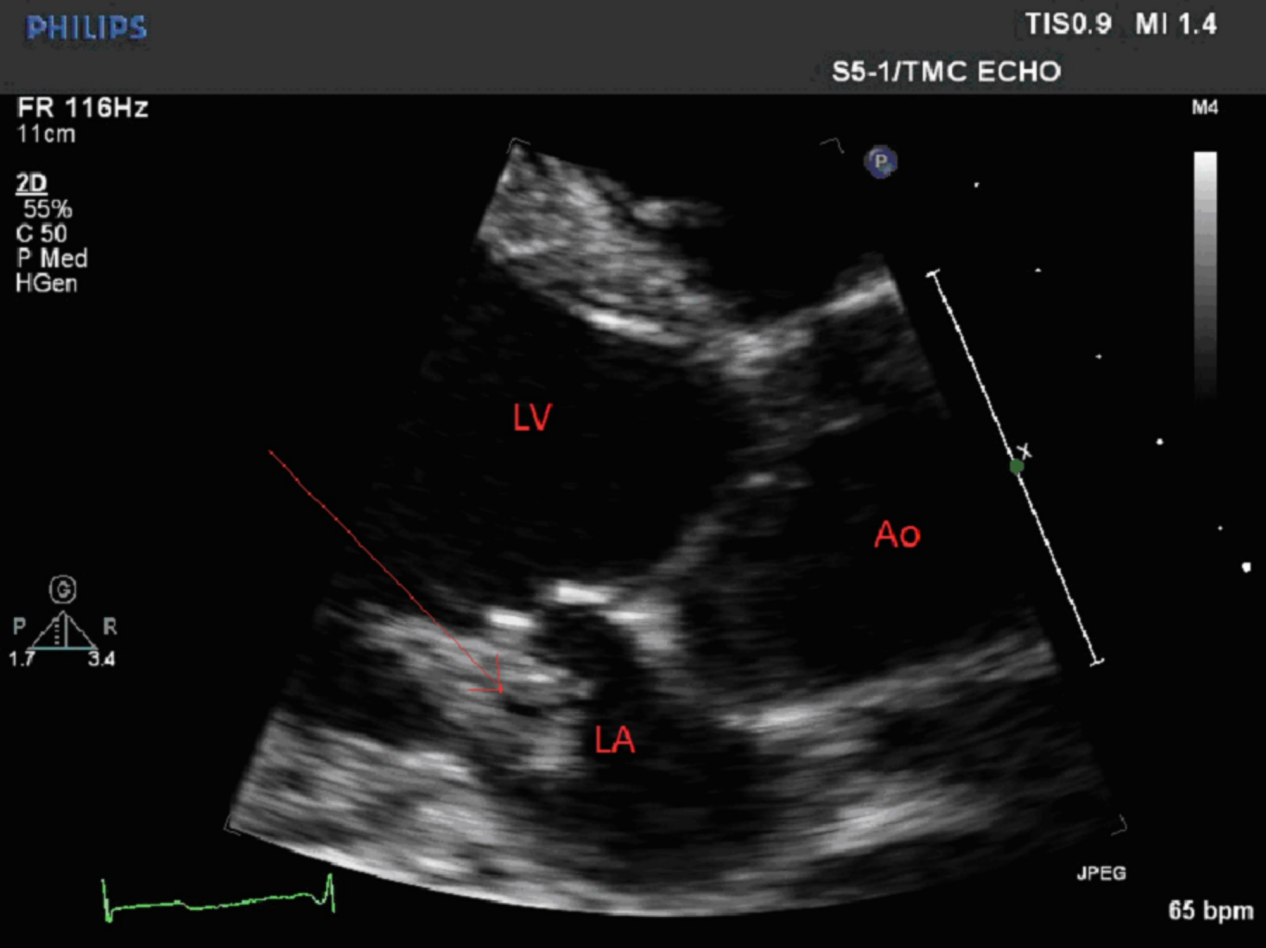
Parasternal long axis view showing the large vegetation (arrow) on the mitral valve. LA: Left Atrium. LV: Left Ventricle. Ao: Aorta.
